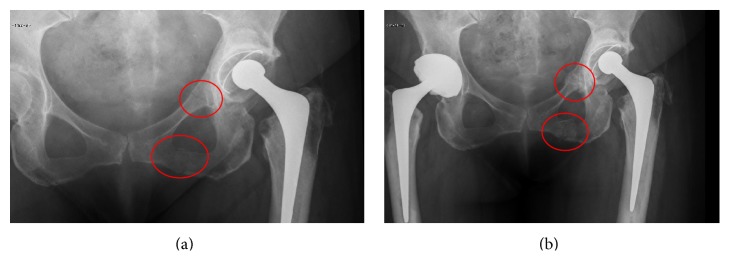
AP radiographs of the pelvis showing (a) bifocal pubic rami fractures at age 58; (b) healing fractures aged 61 (3 years later).
